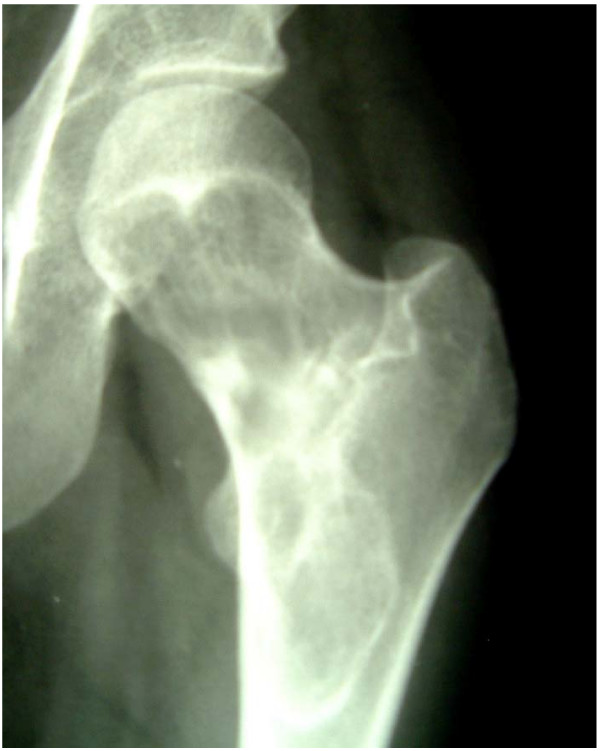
X-Ray, preoperative: Cystic lesion of the proximal femur.
